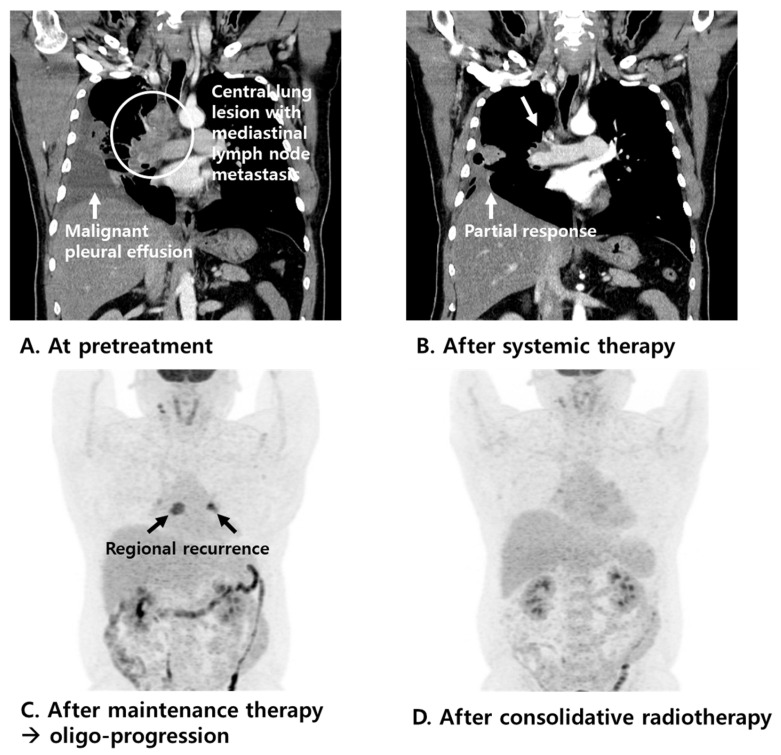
Chest CT images (A) at pretreatment and (B) after systemic therapy (atezolizumab plus etoposide plus carboplatin) in a patient with extensive-stage SCLC who showed partial response on tumor response evaluation. PET-CT images (C) after atezolizumab maintenance therapy and (D) after consolidative radiotherapy.
